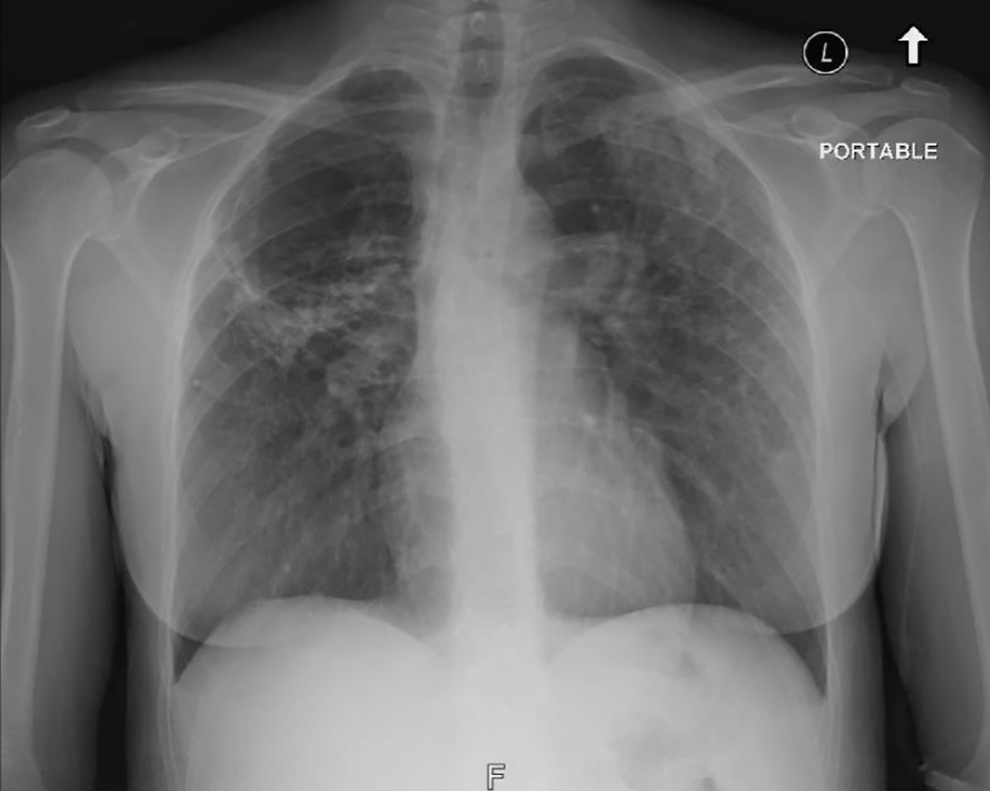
Chest X-ray demonstrating patchy interstitial opacities in bilateral upper lobes and a crescent sign in the left upper lobe.
